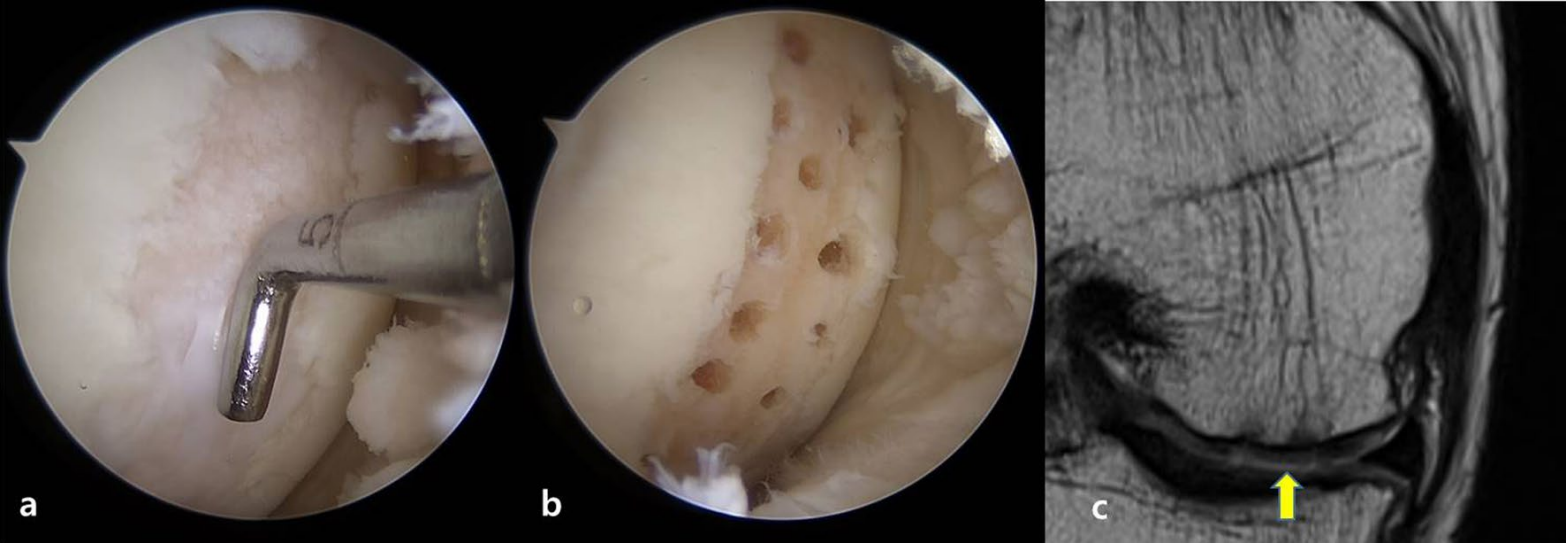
Images of a cartilage defect of the medial femoral condyle a, b after microfracture was performed, and c at 1-year follow-up via magnetic resonance imaging are shown. The yellow arrow indicates repaired tissue
